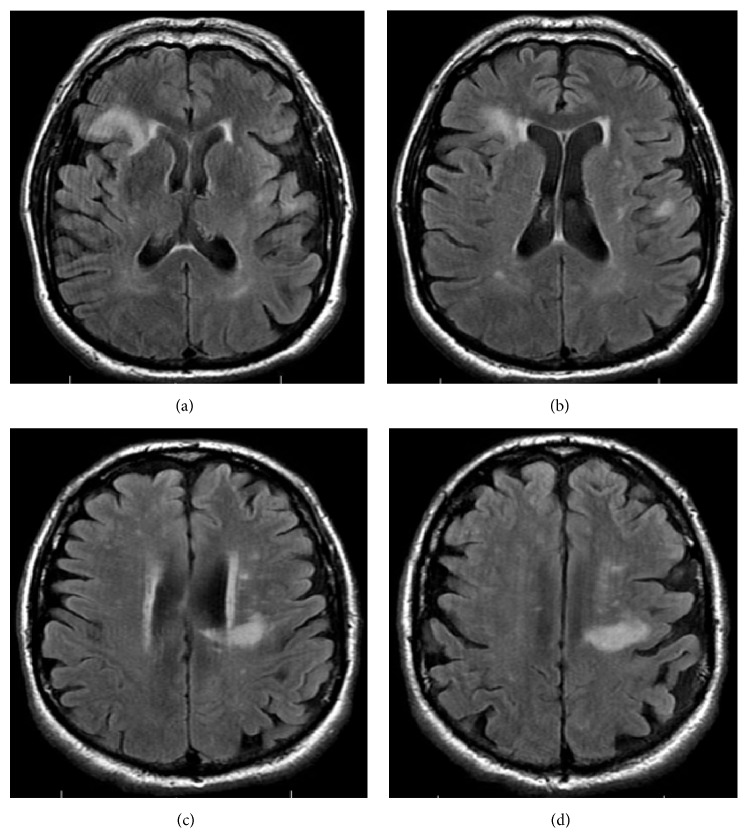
Axial images of MRI of the brain showing multiple confluent foci of T2-weighted FLAIR hyperintensity involving the inferior right and left frontal lobes, as well as periventricular regions (a-b). Hyperintensities are also seen in the left corona radiata (c-d). These areas are representative CNS demyelination. Several foci had restricted diffusion. There was no flow-limiting stenosis, occlusion, or aneurysm in intracranial and extracranial circulations. Images progress superiorly to inferiorly from (a) through (d).
